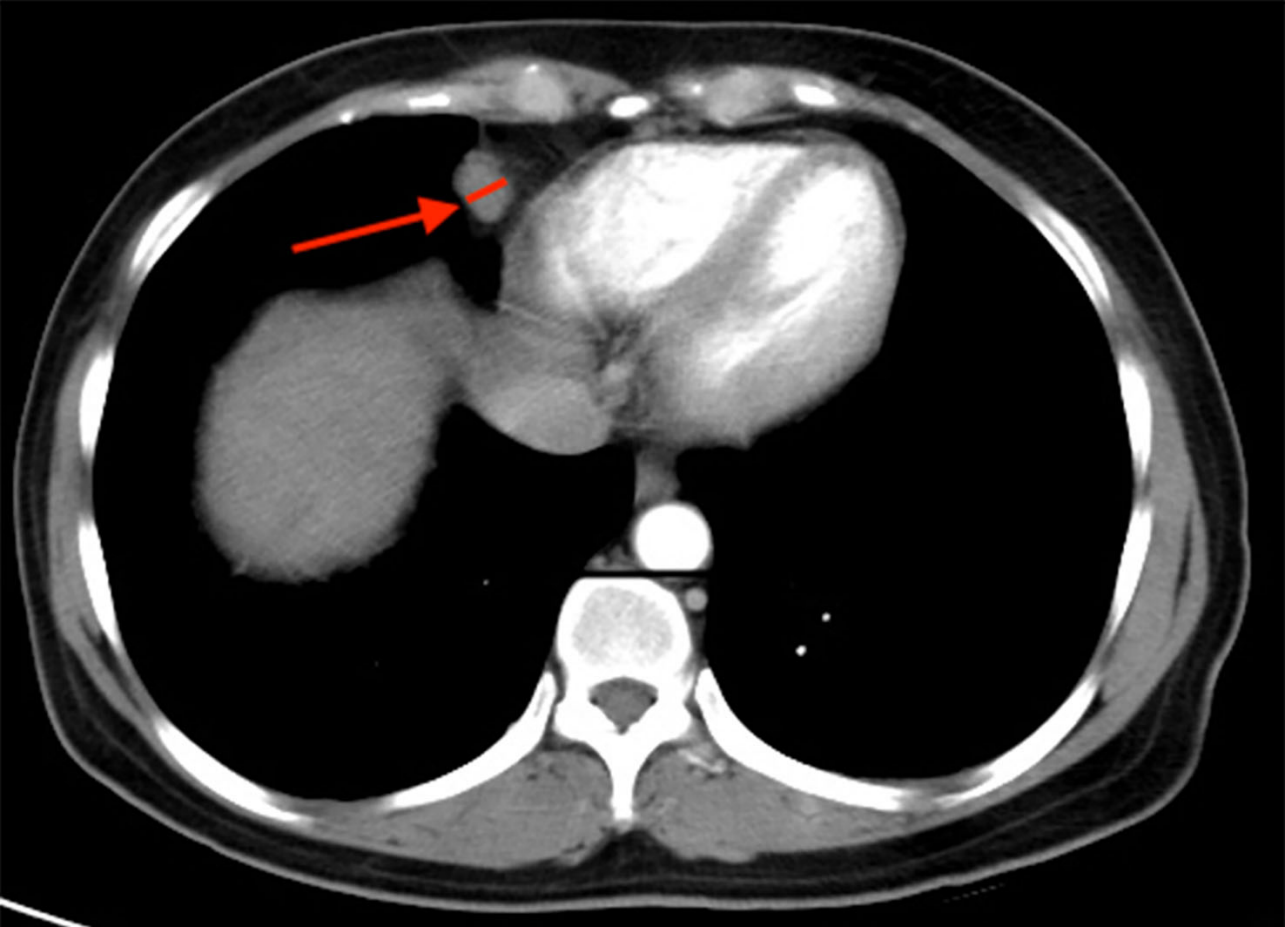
Staging CT image showing an example of cardiophrenic lymph node (CPLN) of 9.4 mm in the short axis and located in the anterior right location.
